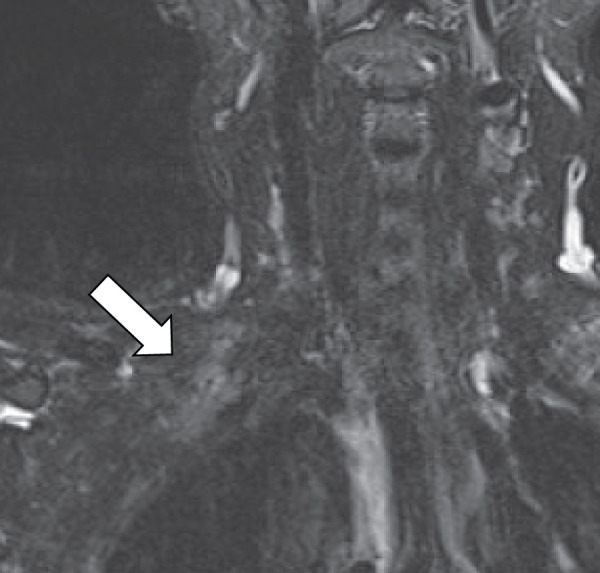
Cervical MRI showing swelling and high signal intensity (white arrow) of the brachial plexus on short tau inversion recovery imaging at the dorsal side of the central right clavicle, consistent with brachial plexus injury.
